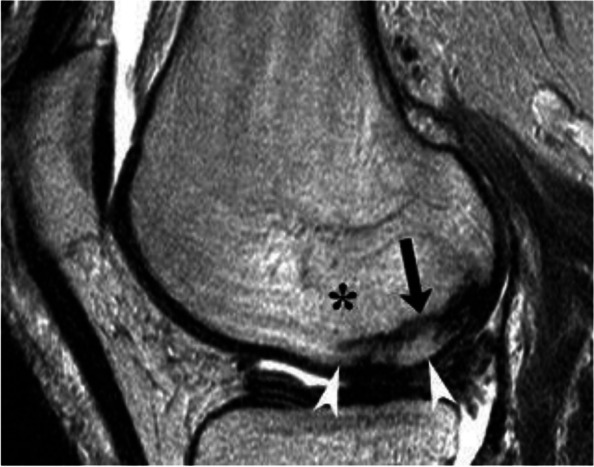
SIF in a patient with osteomalacia. T2-weighted MR image shows a low signal intensity band corresponding to a particularly thick trabecular fracture (arrow). The bone marrow of this portion of the condyle shows BME-like high signal intensity (asterisk). Note that the BME-like signal extends between the fracture and the bone-cartilage interface (arrowheads). That area of the subchondral bone is in continuity with the rest of the epiphyseal area
